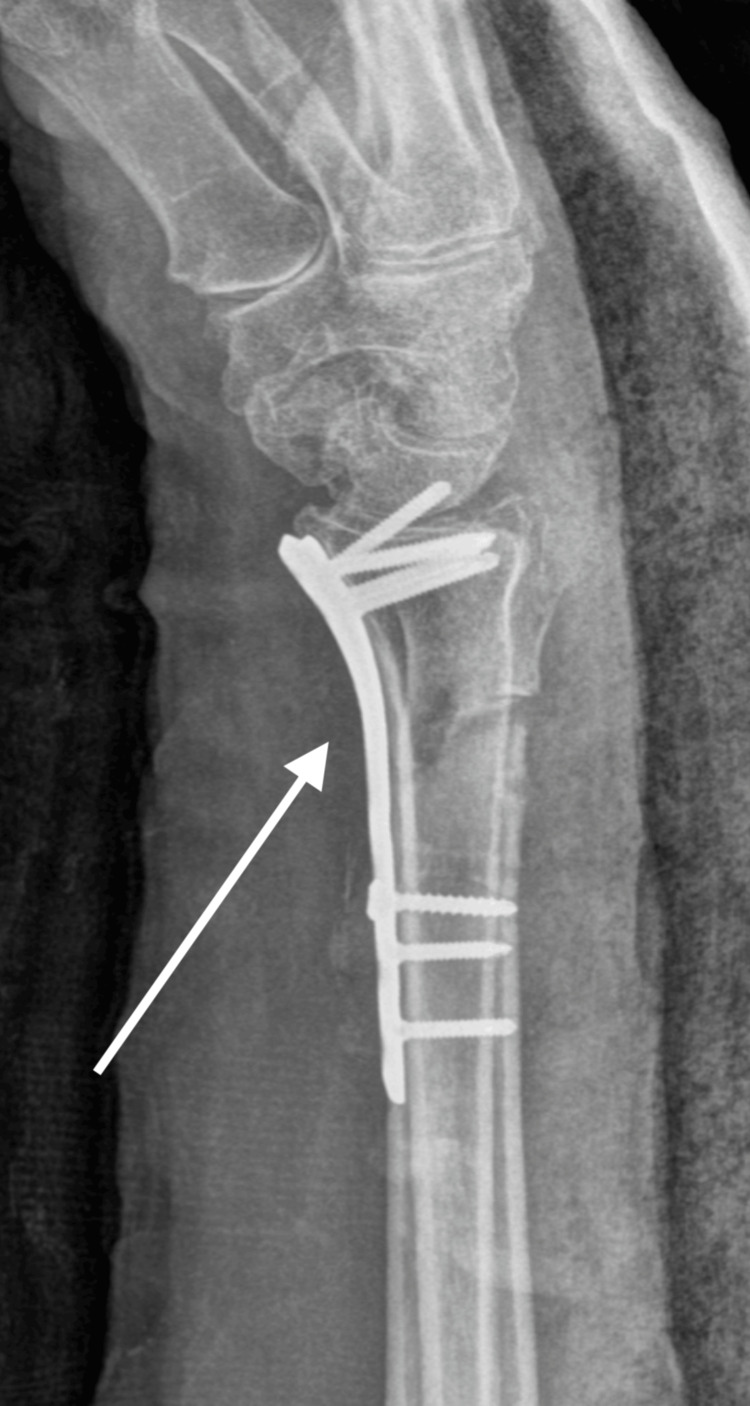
X-ray after bended plate removal and new plate insertion, lateral view
